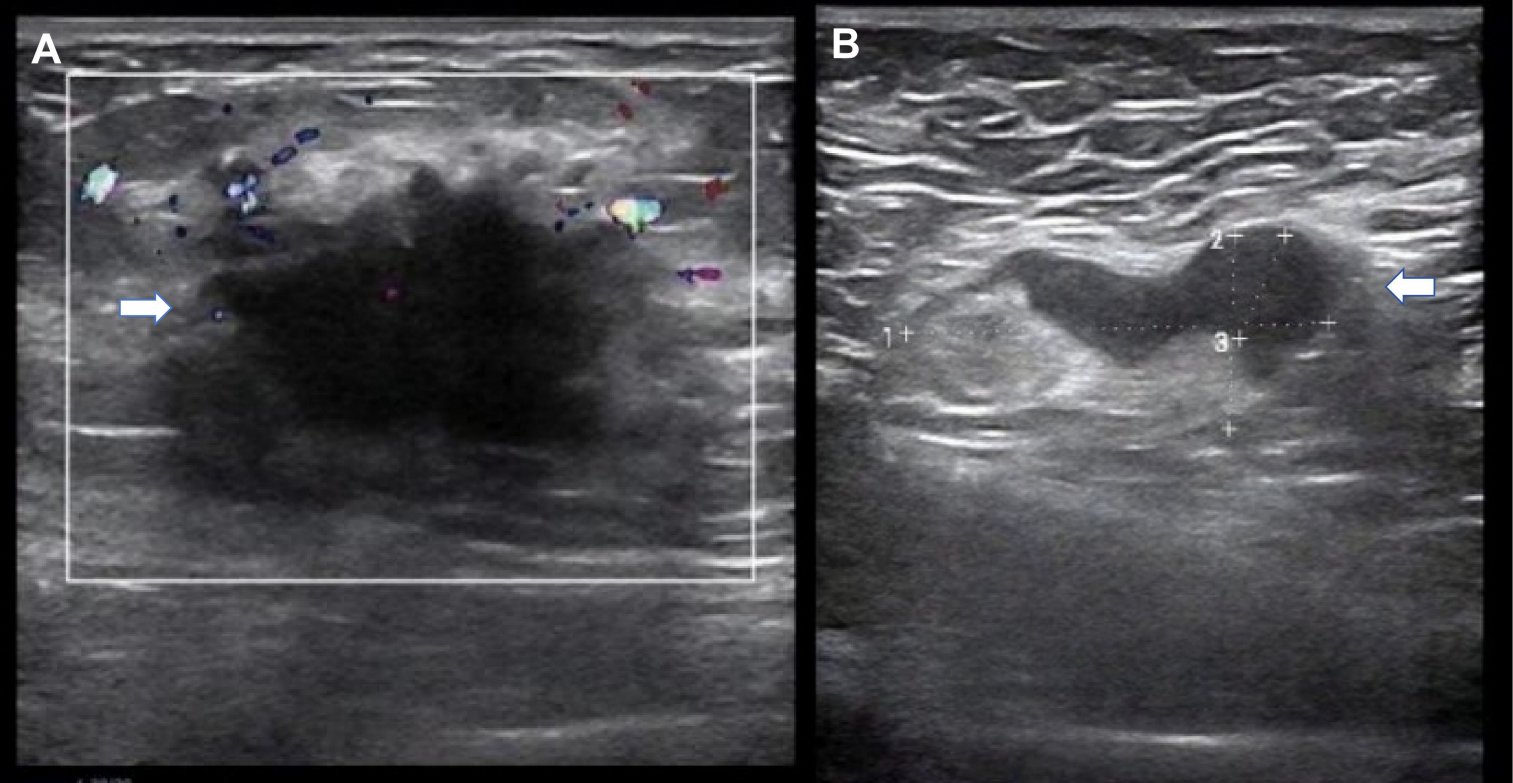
Ultrasound images of the right breast and axilla at diagnosis (16 weeks of gestation). (A) The arrow indicates a hypoechoic, irregular mass located in the upper outer quadrant of the right breast, measuring 3.2×2.1 cm. (B) The arrow points to an enlarged right axillary lymph node measuring 3.4 cm in maximum diameter, showing cortical thickening suggestive of metastasis.
